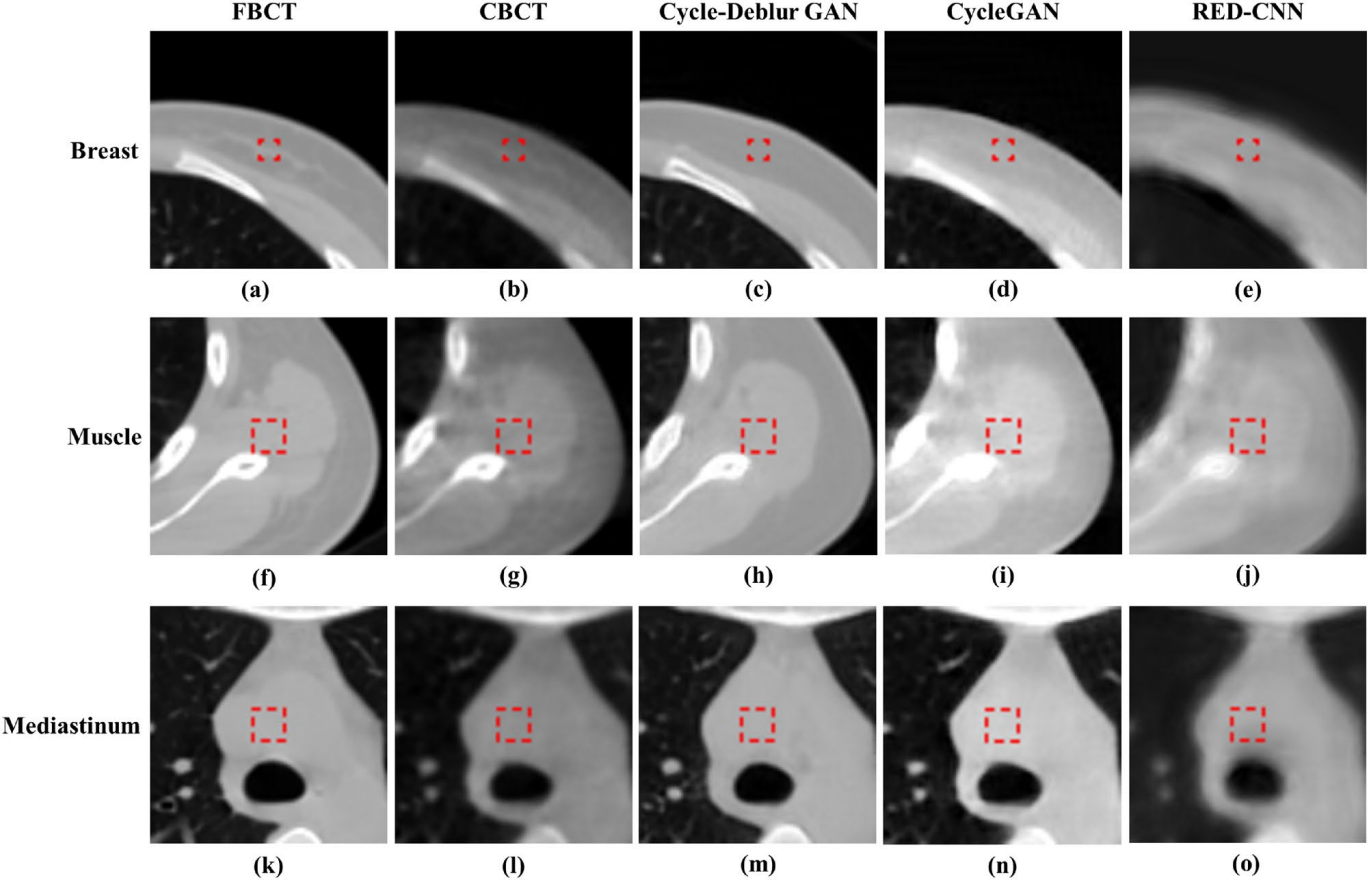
The soft tissue ROIs of the breast, muscle and mediastinum for quantitative evaluation are shown in (a,f,k): FBCT, (b,g,l): CBCT, (c,h,m): Cycle-Deblur GAN, (d,i,n): CycleGAN and (e,j,o): RED-CNN were performed by XVI software and PMOD software. (W = 1450, L = − 225 for all CT images).
